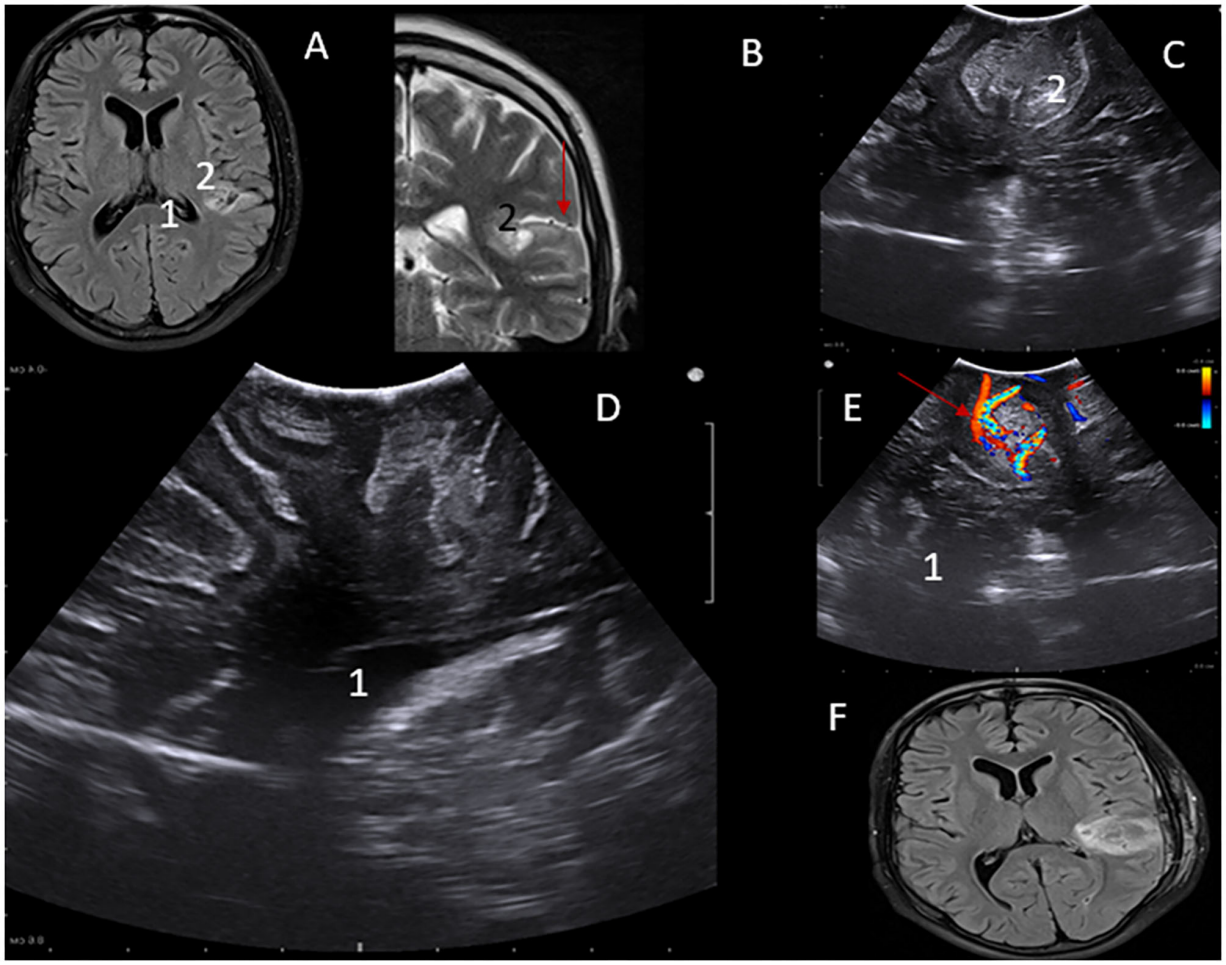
(A) MRI – flair axial view of Lt parital lobe lesion- 1. (B) MRI – T2WI MRI coronal view of Lt parietal lobe lesion- 2. iUS depiction of low-grade lesion in axial. (C,D) Saggital views Perilesional vascular structures can be visualized using Color Doppler function-Red arrow (A,E). Post-operative MRI showing gross total resection of the lesion (F).
